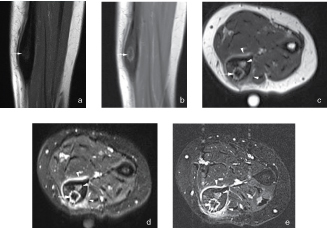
(a) Coronal *T*_1_ weighted image. (b) Coronal *T*_2_ weighted image. (c) Axial *T*_2_ weighted image. (d) Axial fat-suppressed *T*_1_ weighted image after administration of gadolinium contrast. (e) Axial subtraction of the images before and after gadolinium contrast administration. MRI shows a focal intracortical lesion in the ulna with low central signal intensity and mineralization on both *T*_1_ and *T*_2_ weighted images (white arrows). After administration of gadolinium contrast, there is marked peripheral enhancement of the lesion with perilesional bone marrow and soft-tissue oedema (white arrowheads).
